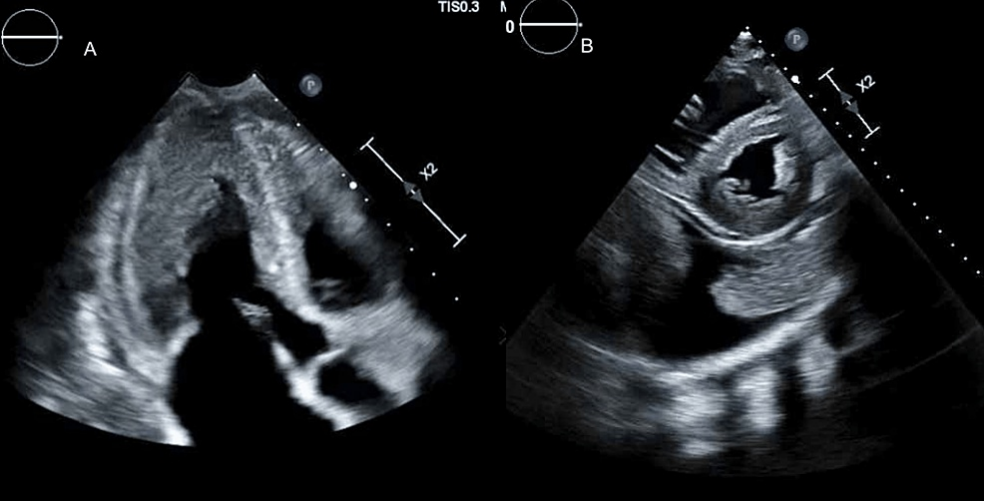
Echocardiography in cardiac amyloidosis A. Showing right ventricular hypertrophy. B. Left ventricular hypertrophy with small pericardial effusion
